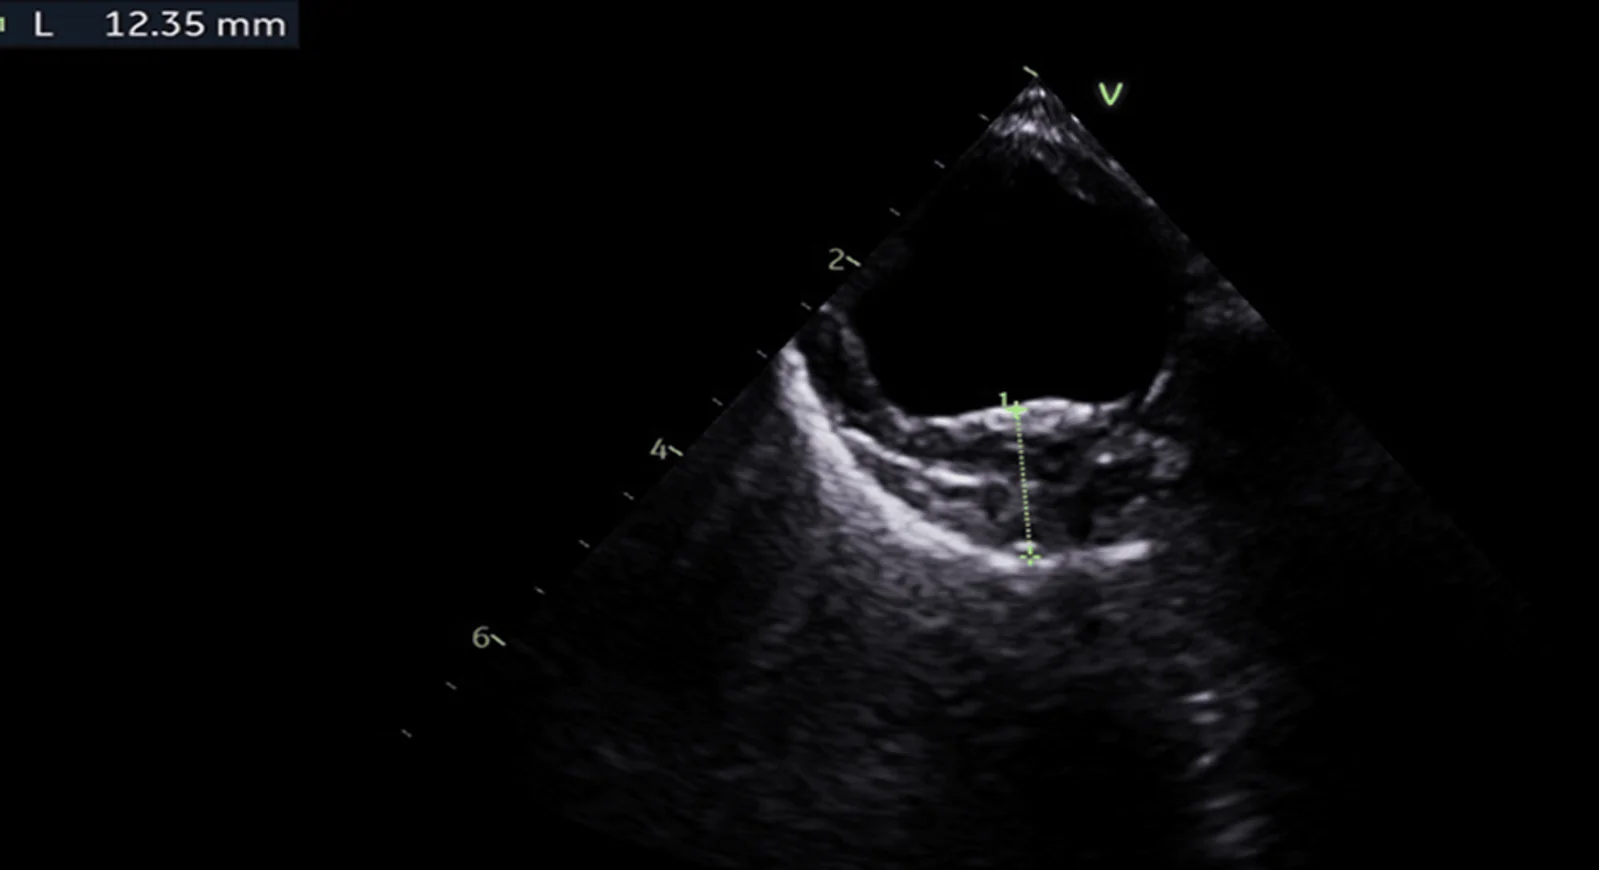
Transverse view of the descending thoracic aorta on transesophageal echocardiography, showing a large irregular mural atheromatous plaque with a maximum thickness of 12.35 mm
